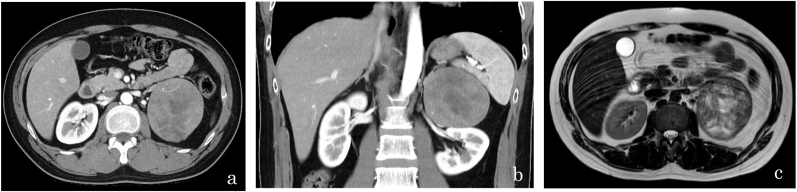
Radiologic findings. (a, b) Contrast-enhanced computed tomography revealing an 8.6 × 7.7-cm heterogeneously enhancing left suprarenal mass with increased vascularity. (c) T2-weighted magnetic resonance imaging showing a hyperintense left adrenal mass.
